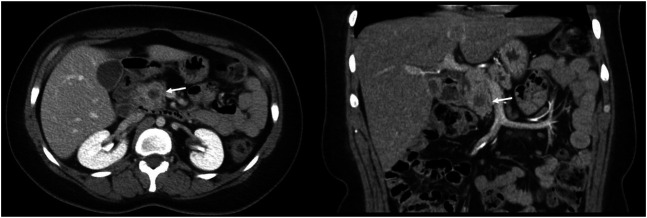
Computed tomography showing a solid 12 mm hypoenhancing mass in the inferior pancreatic head with associated pancreatic ductal dilation (5 mm) and mild hepatic steatosis.
